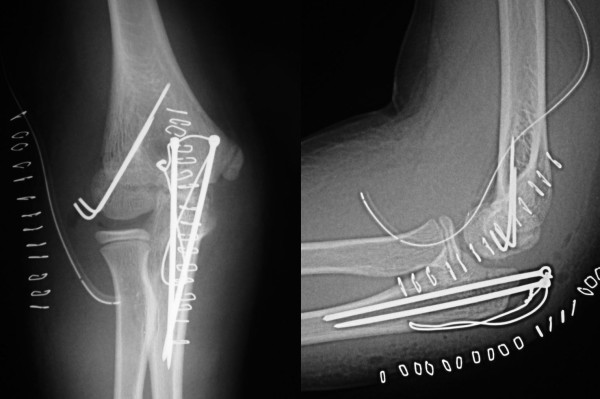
Postoperative anteroposterior (A) and lateral (B) radiographs of the elbow.
